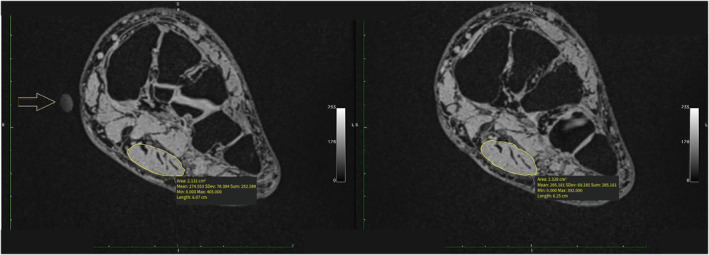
MR image depicting a slice showing gel capsule at maximum size (left) and image depicting no capsule (right).
